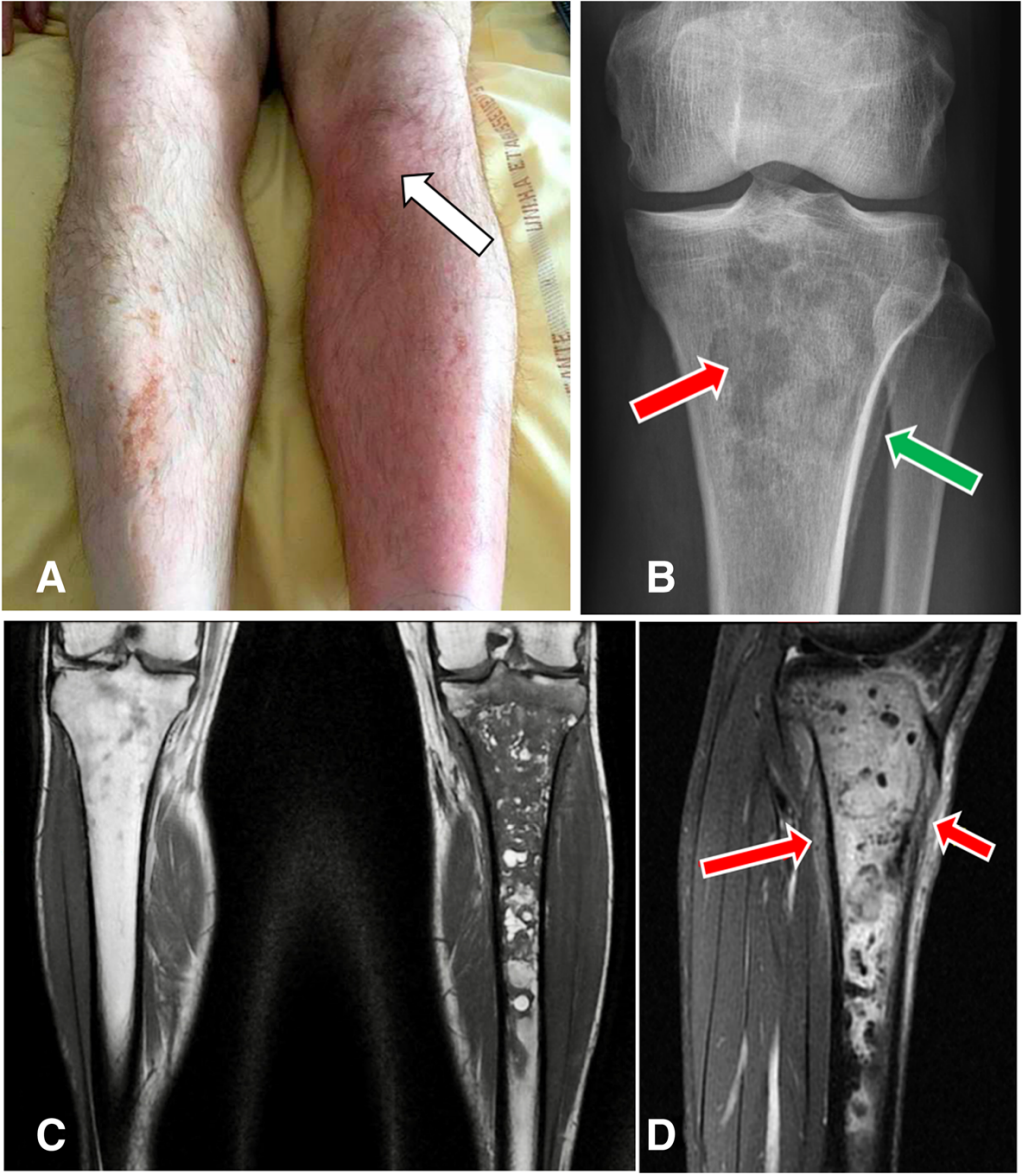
a Image of the left tibia, well-defined area with redness, warmth and swelling over his left proximal tibia corresponding to the site of the intraosseous injection (arrow). The contralateral leg is normal. b X-rays of left proximal tibia in front view with multiple ill-defined lytic lesion in the metaphysis and the epiphysis and geographic pattern (red arrow) and also proximal tibial blurred periosteal appositions (green arrow). c Coronal T1-weighted section with massive epiphyso-metaphyseal hypoT1 intra-medullary bone edema extended to the diaphysis up to the middle third of the leg. d Sagittal fat-sat T2–weighted section with significant hyperT2 infiltration of soft tissues (arrows)
